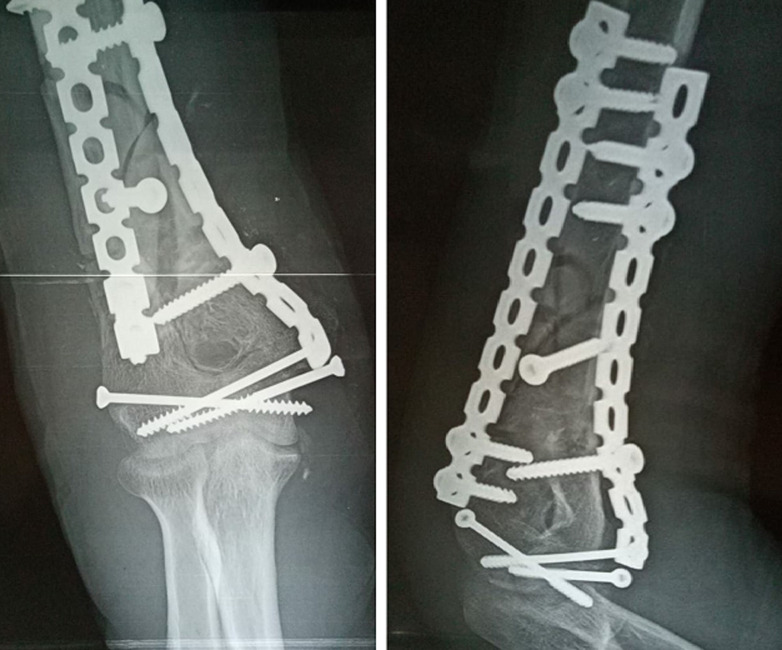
radiographie de contrôle post-opératoire montrant un montage orthogonal: une plaque postéro-latérale et une plaque médiale pour une fracture type C2
